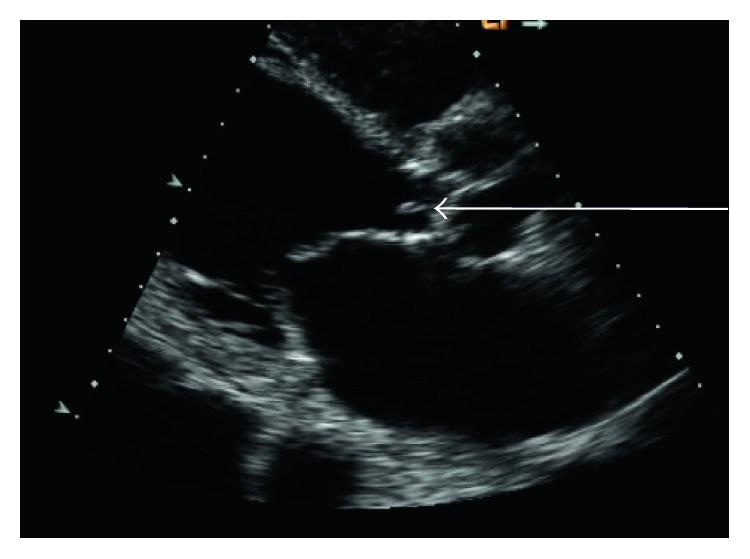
Transthoracic echocardiogram showing vegetation at the valley of the aortic valve cusp.
